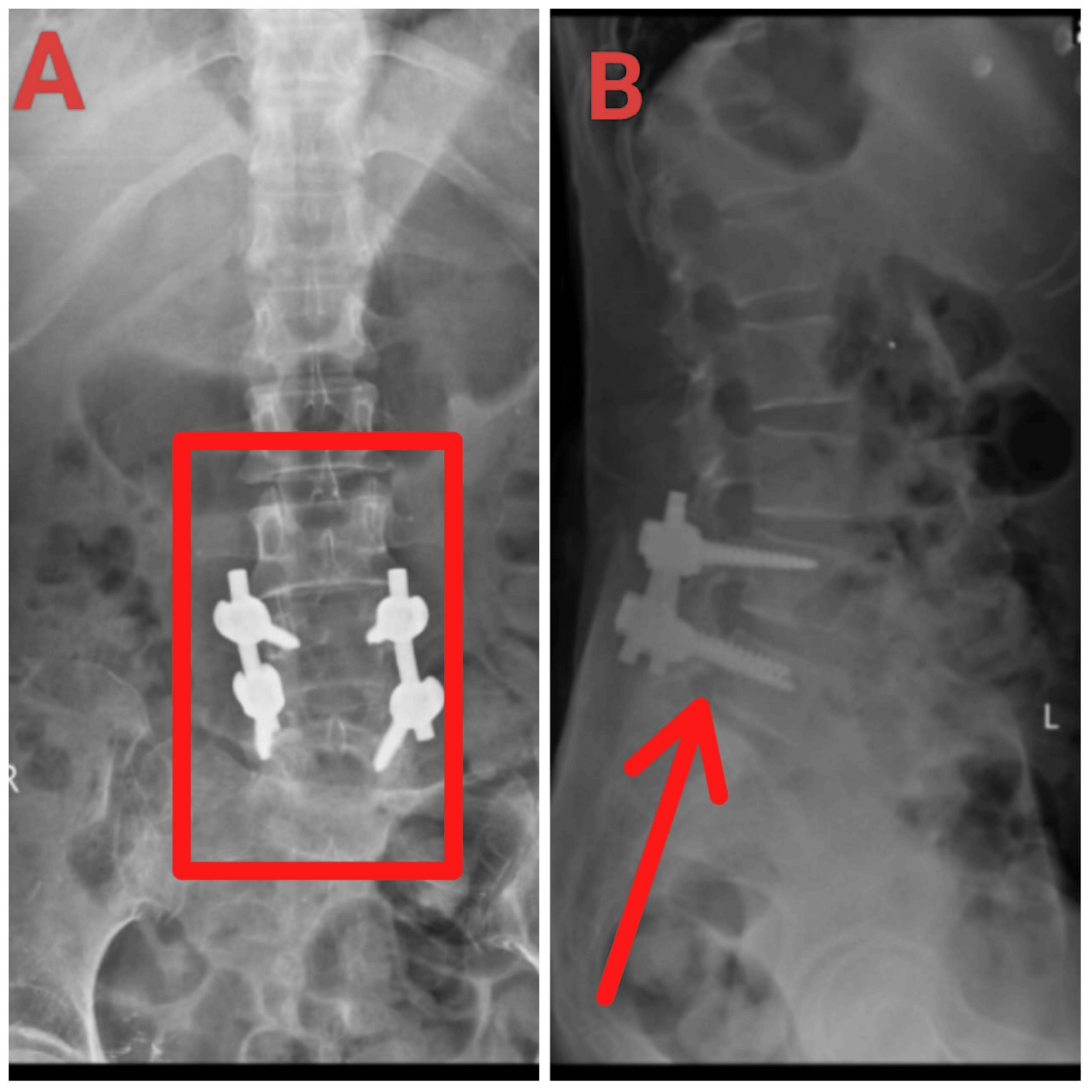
Postsurgery X-ray image (A) Anteroposterior (AP) view: The AP view complements this by offering a frontal perspective, showing the symmetric alignment of the screws and rods, indicating proper surgical placement. (B) Lateral view: The lateral view shows the pedicle screw and rod fixation hardware at the L4-L5 level, providing a side view that allows for the evaluation of spinal alignment and hardware placement in the sagittal plane
